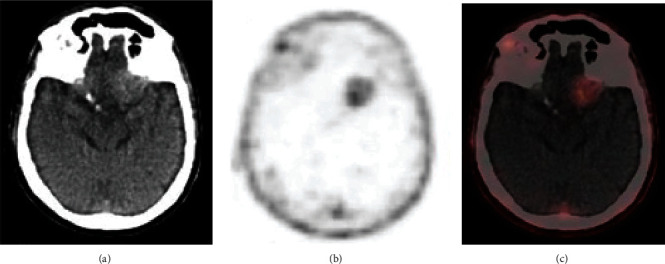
^68^Ga-PSMA PET/CT in a representative meningioma. (a) T1-weighted MRI. (b) Head CT. (c) ^68^Ga-PSMA PET/CT. Reproduced with permission from Figure 2 of Bilgin et al. [73].
